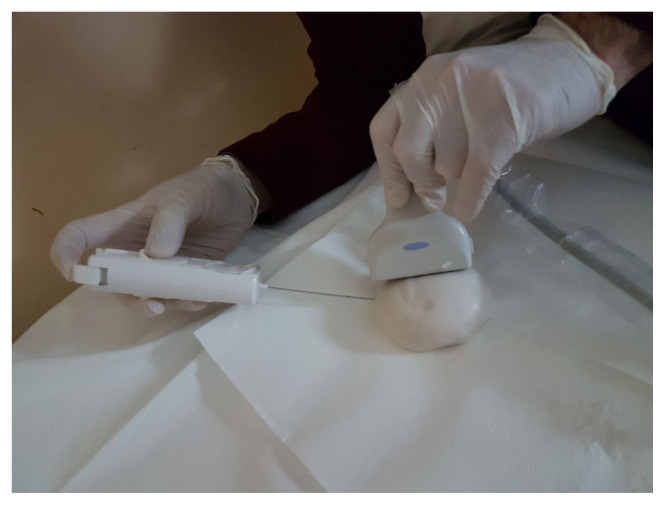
Ultrasound-guided needle biopsy of SH-P.
